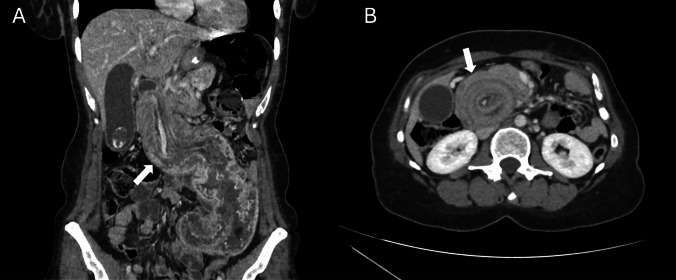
Frontal view of abdominal computed tomography scan revealing remnant stomach intussusception through the duodenum (white arrow, A). Axial view of abdominal computed tomography scan showing a « target sign» (white arrow, B)
